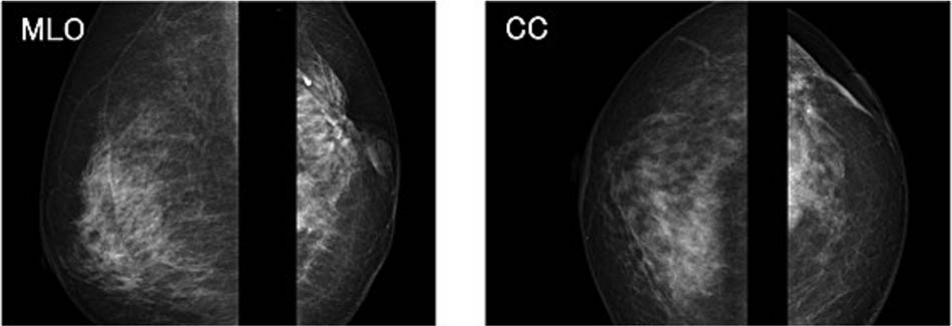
Chest radiograph (frontal view). No obvious abnormal findings were observed in the right breast.
